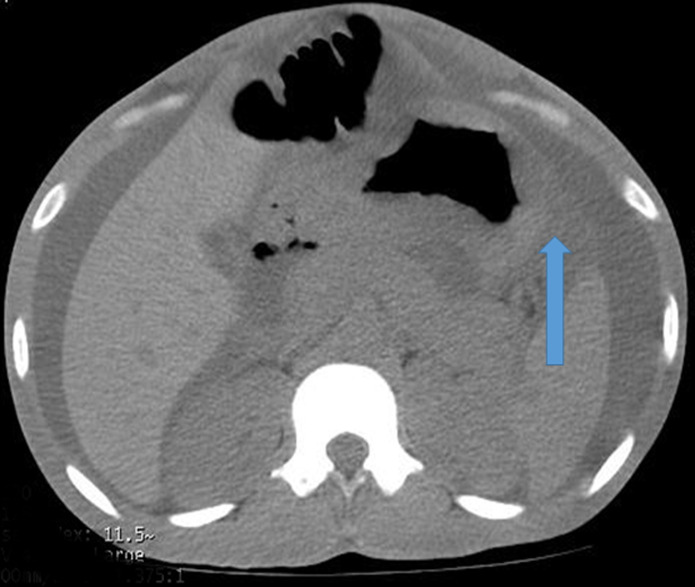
axial view of abdominal CT scan showing gastric wall thickening; differential, Linitis plastica
